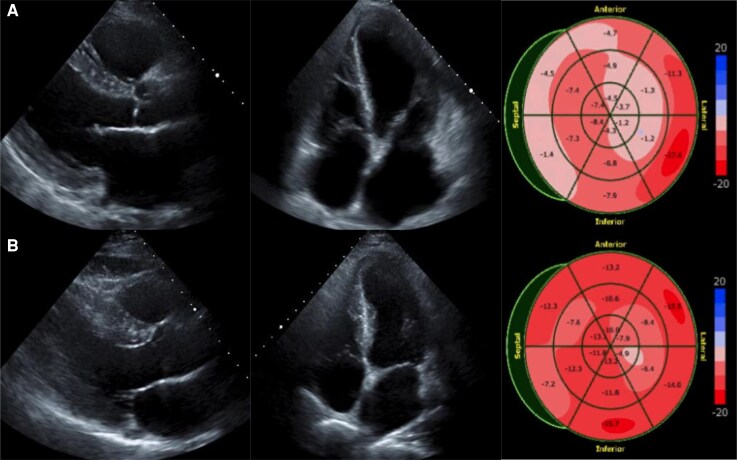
Echocardiographic changes before and after percutaneous coronary intervention. (*A*) Pre-intervention echocardiography. Left ventricular ejection fraction was reduced to 24%, with markedly decreased strain particularly in the left anterior descending artery territory. (*B*) Post-intervention echocardiography. Left ventricular ejection fraction recovered to 46% with wall motion recovery in the left anterior descending artery territory.
